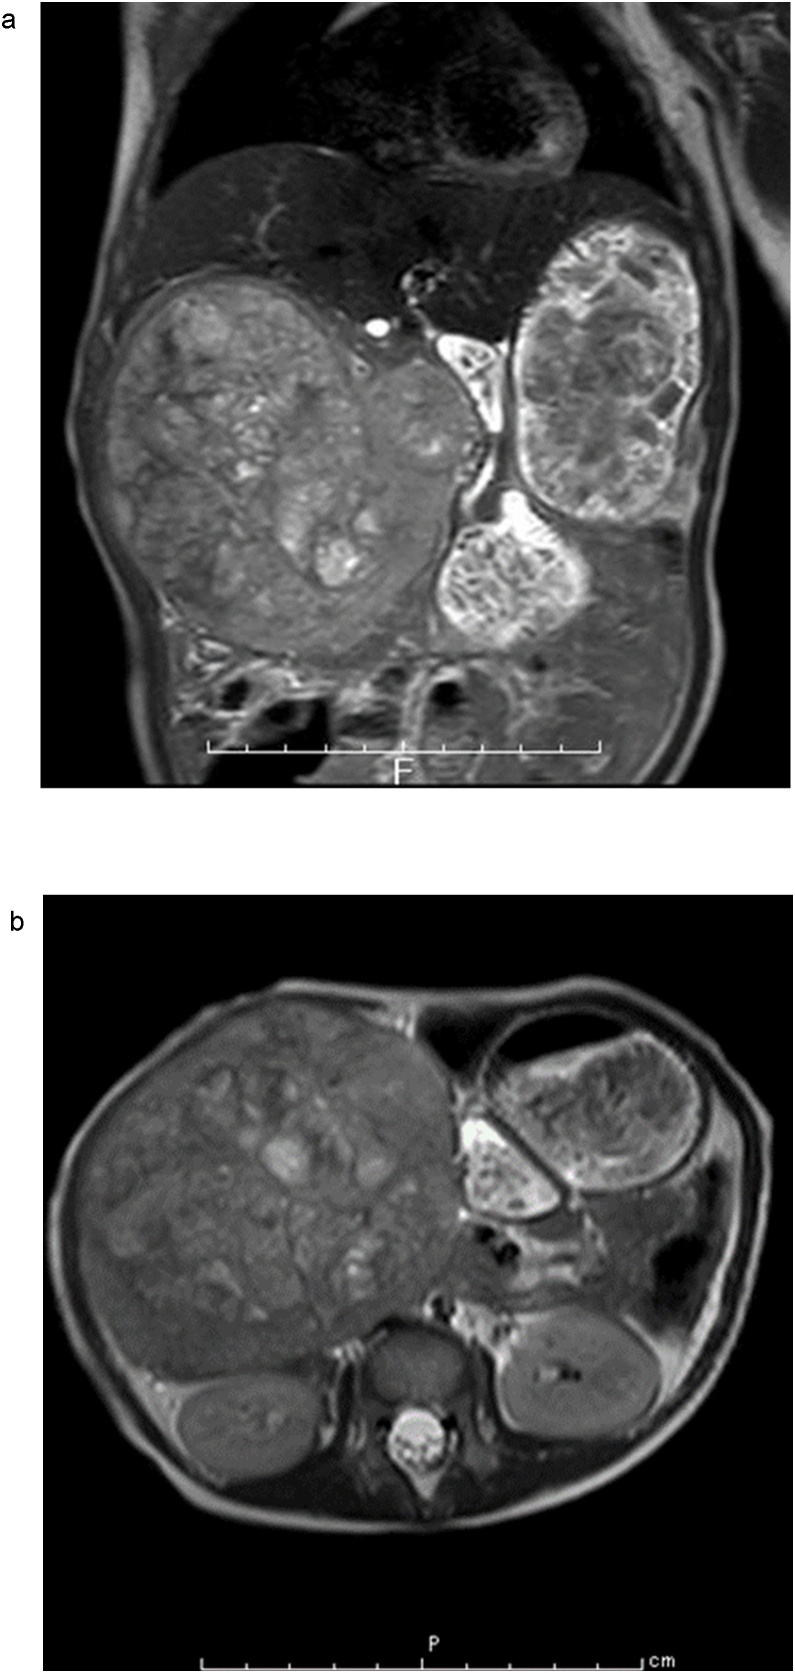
a,b: Abdominal magnetic resonance imaging (T2weighted) showing a large solid tumor approximately 100 mm in diameter protruding into the abdominal cavity from the right hepatic lobe.
